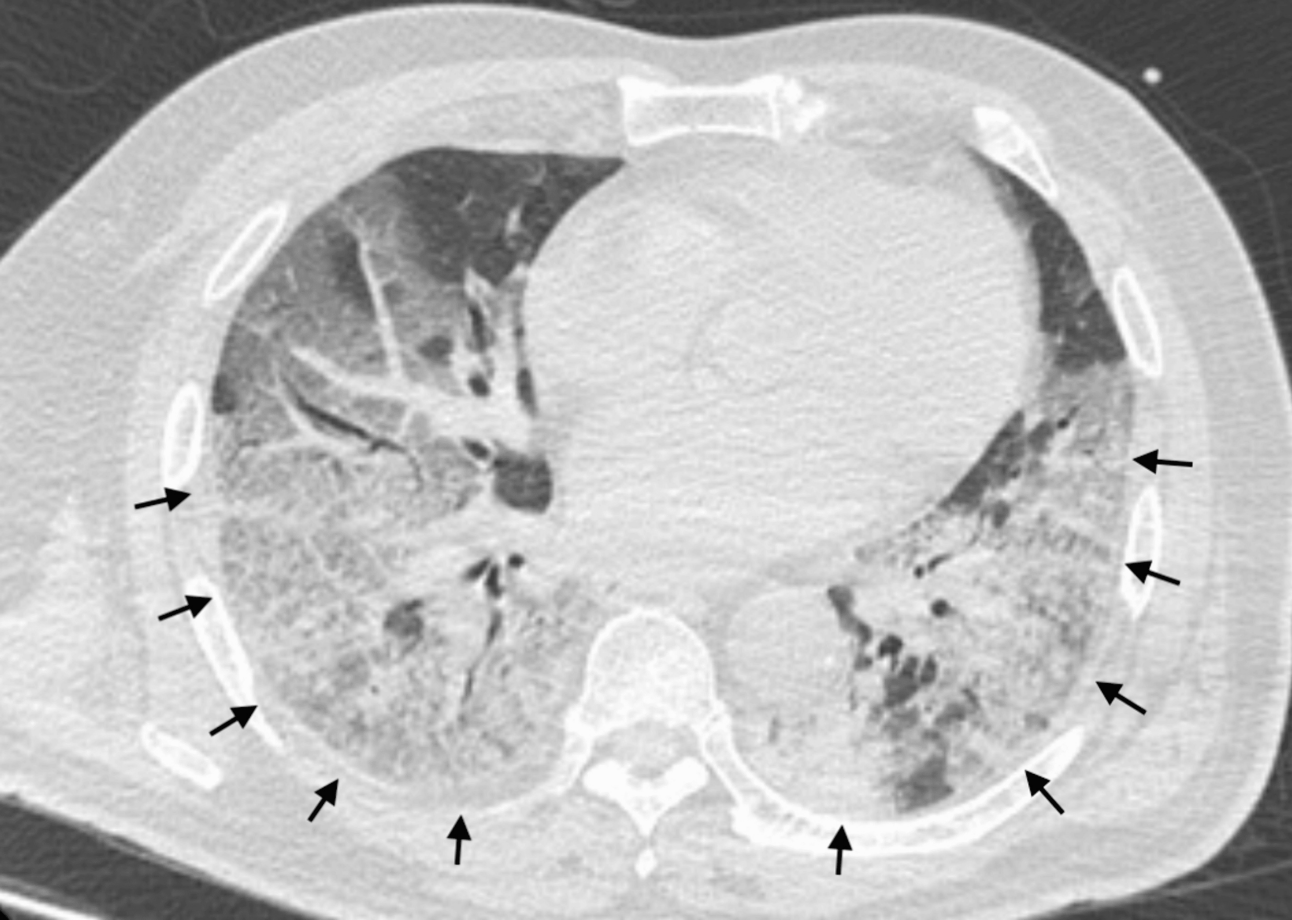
Chest computed tomography from the referral hospital Computed tomography revealed extensive ground-glass opacities, predominantly in the periphery of both lungs.
